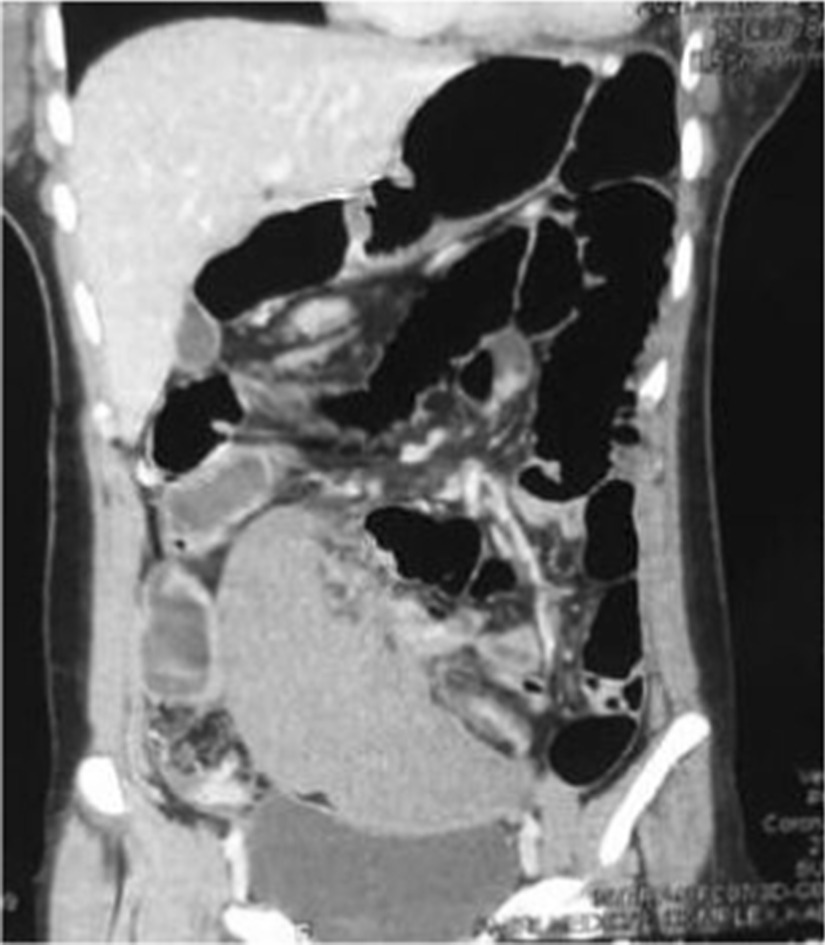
A coronal CT section showing empty left hemidiaphragm distended small and large bowel, misplaced non enhanced mildly enlarge spleen in the right lower abdomen extending into the pelvis, resting above the urinary bladder and bright capsule (rim sign) of the surface adjacent to the bladder
